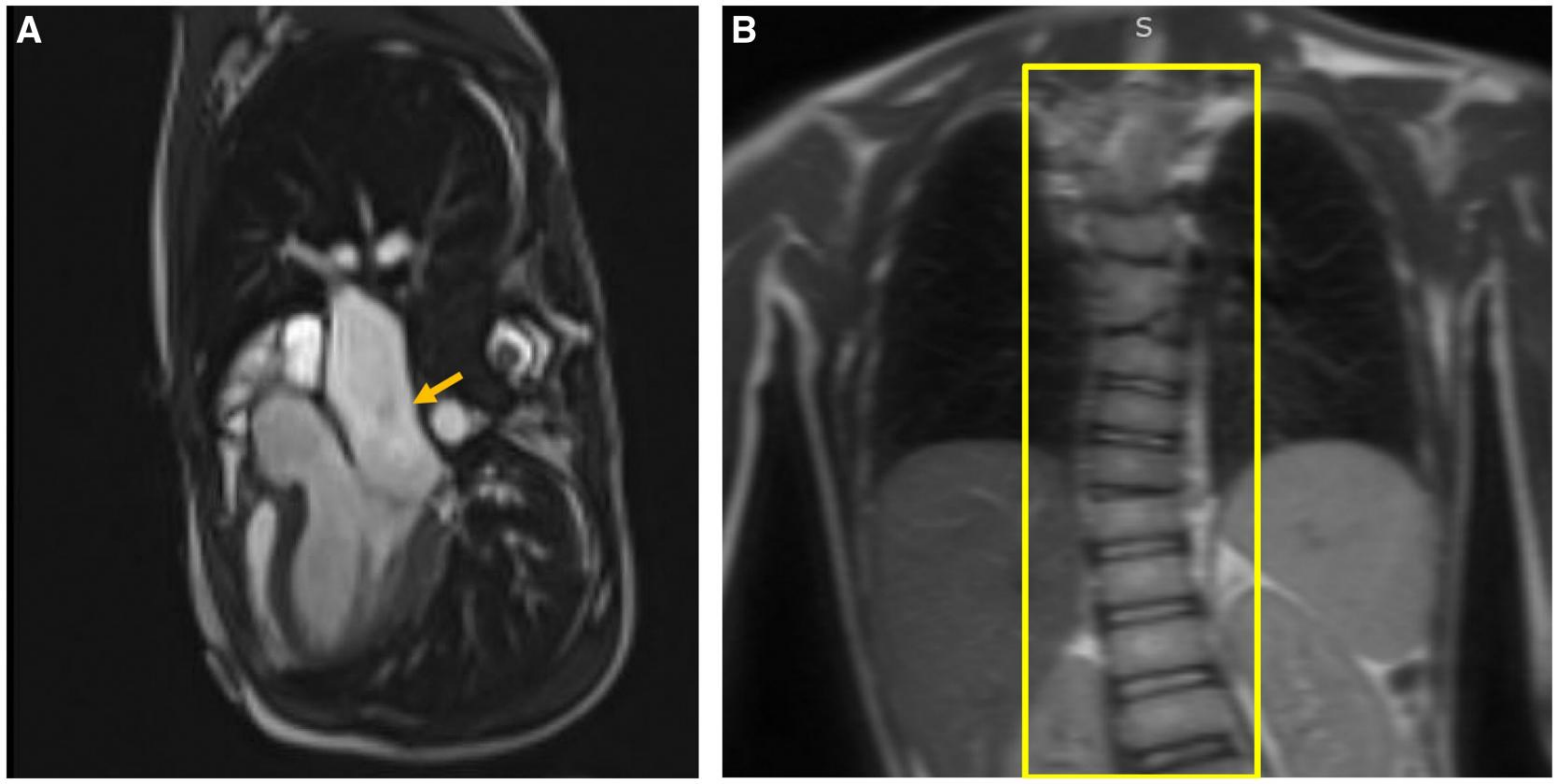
A, MRI Cardiac Morphology with and without contrast 3-chamber long axis view, yellow arrow shows aortic root dilation. B, Cardiac MRI coronal thorax view, yellow box displays dextroconvex scoliosis of the thoracolumbar junction, and multilevel malformations of the thoracic spine segmentation.
